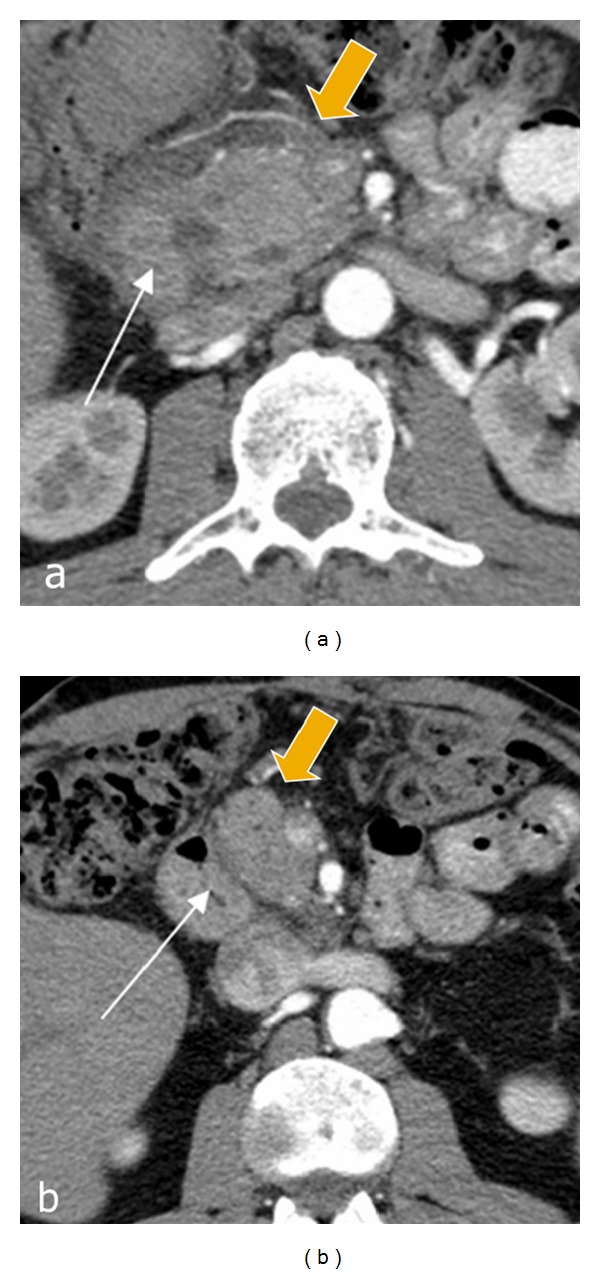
Duodenal dystrophy with moderate chronic orthotopic pancreatitis. Patient, 32 y.o. Arterial phase of multidetector computed tomography before (a) and 6 months after (b) the pancreas-preserving resection of the second portion of the duodenum with the jejunal interposition. (a) There is a septated cystic structure (thin arrow) in the medial duodenal wall with prominent inflammation and fibrosis around the duodenum and pancreatic head (thick arrow). (b) Neoduodenum (thin arrow) and pancreatic head (thick arrow) without signs of inflammation or fibrosis.
